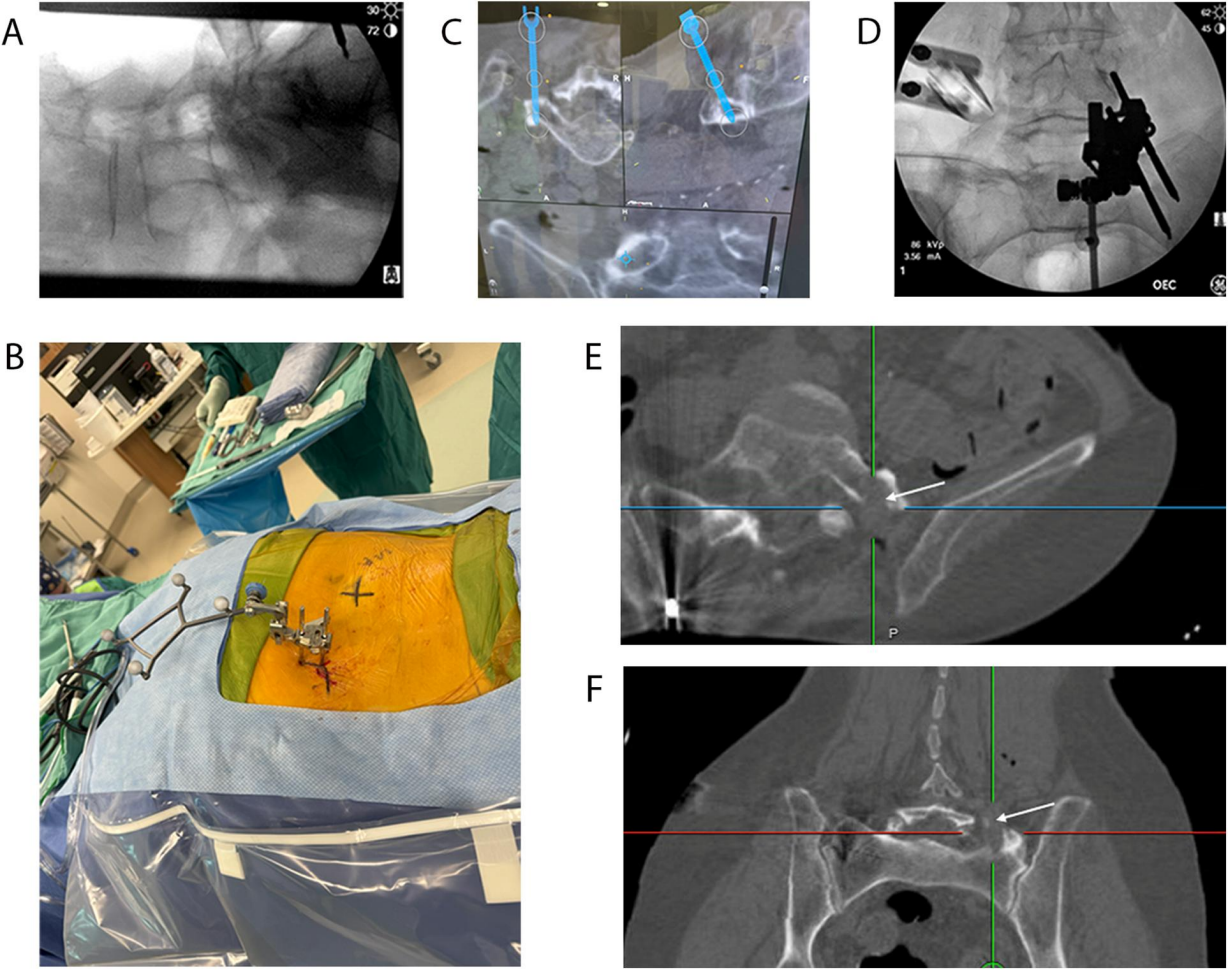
Intraoperative localization and navigation. Lateral intraoperative X-ray (A) indicating the position of the brain lab fiducial marker in the superior iliac spine (ASIS). The intraoperative image demonstrates the location of the BrainLab fiducial marker in the ASIS and the left-sided skin incision site (marked with a black “X”). Intraoperative brain lab navigation demonstrating live axial, sagittal, and coronal views with the trajectory (C) used to dock the spine tube system directly onto the left anomalous L5 TP. Anterior–posterior intraoperative X-ray (D) demonstrating the BrainLab fiducial positioned in the ASIS and the spine tube system after insertion. Intraoperative CT obtained after left anomalous TP resection demonstrating completed processsectomy and removal of pseudoarthrotic site in the axial (E) and coronal (F) planes.
